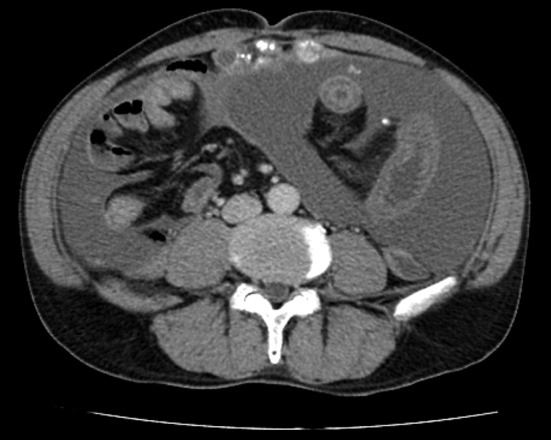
Abdominal computed tomographic scan before the initial operation showing massive ascites and peritoneal thickening with coarse calcifications.
